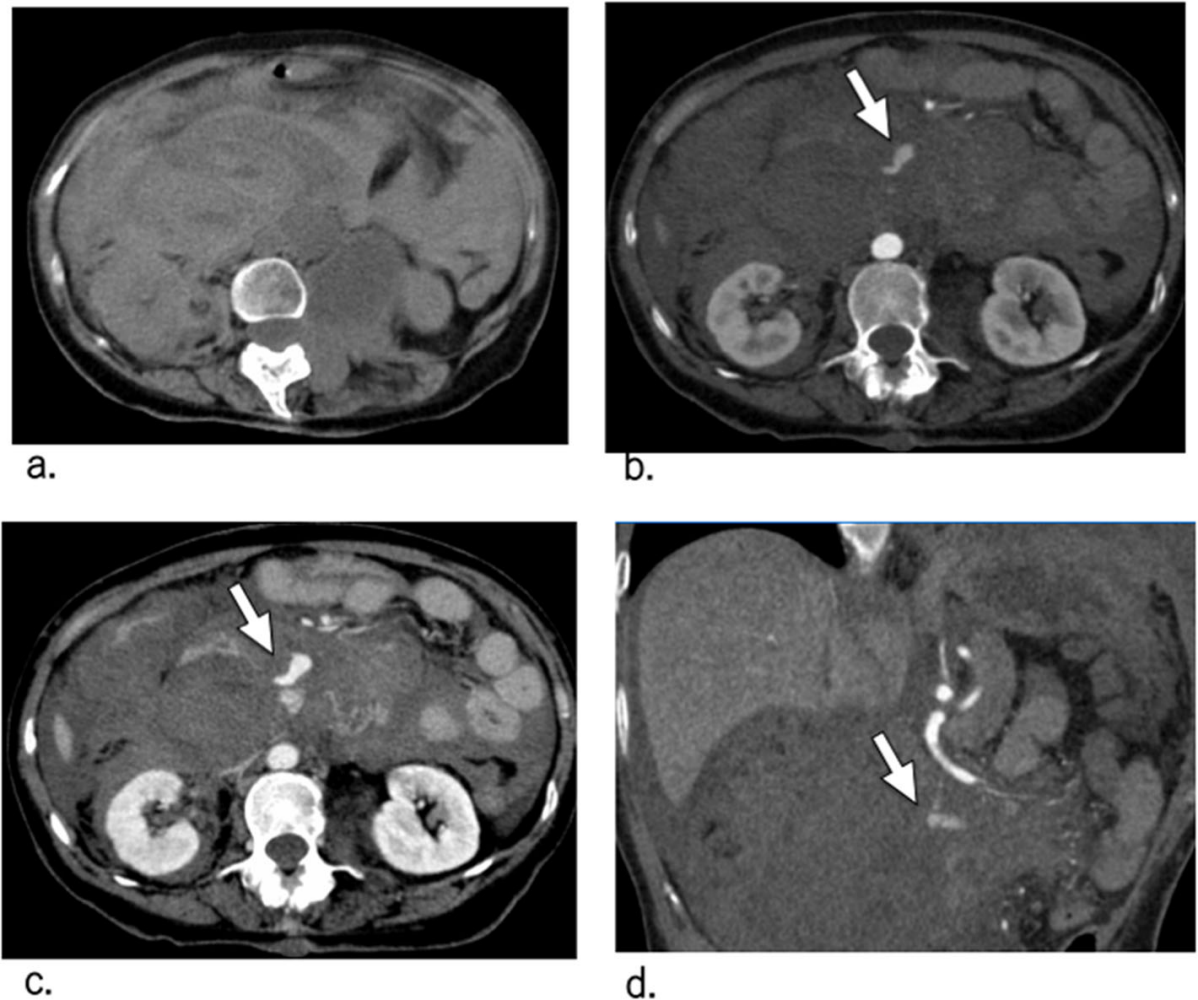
Computed tomography (CT) at symptom presentation. a Non-contrast-enhanced CT image showing a large retroperitoneal hematoma around the duodenum and haemorrhagic ascites. b Contrast-enhanced CT arterial phase image showing a large retroperitoneal hematoma with definite pseudoaneurysm (white arrow). c Contrast-enhanced CT portal venous phase image showing a large retroperitoneal hematoma with definite pseudoaneurysm (white arrow). d Contrast-enhanced CT arterial phase coronal images (slab-maximum intensity projection) showing the superior mesenteric artery (SMA) and pseudoaneurysm
